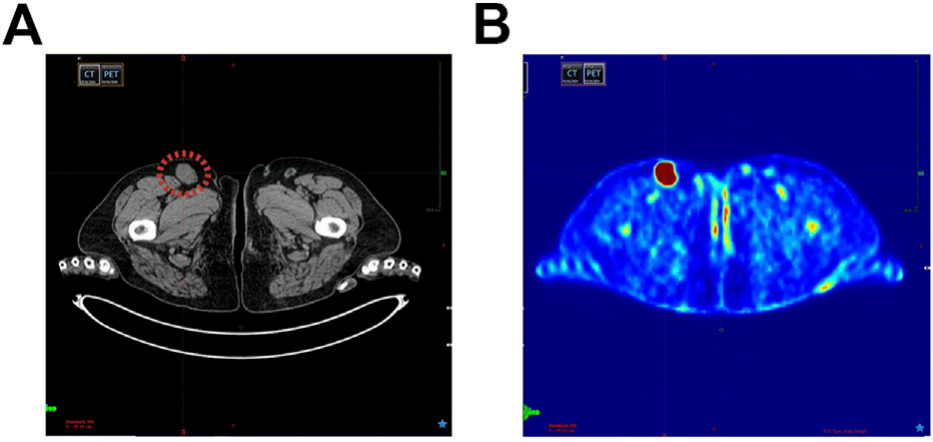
(A) Metastatic groin lymph node (marked in red) in a patient diagnosed with soft tissue sarcoma (STS), computed tomography (CT) image. (B) The same patient, lymph node metastases shown by positron emission tomography-computed tomography scan (PET-CT).
